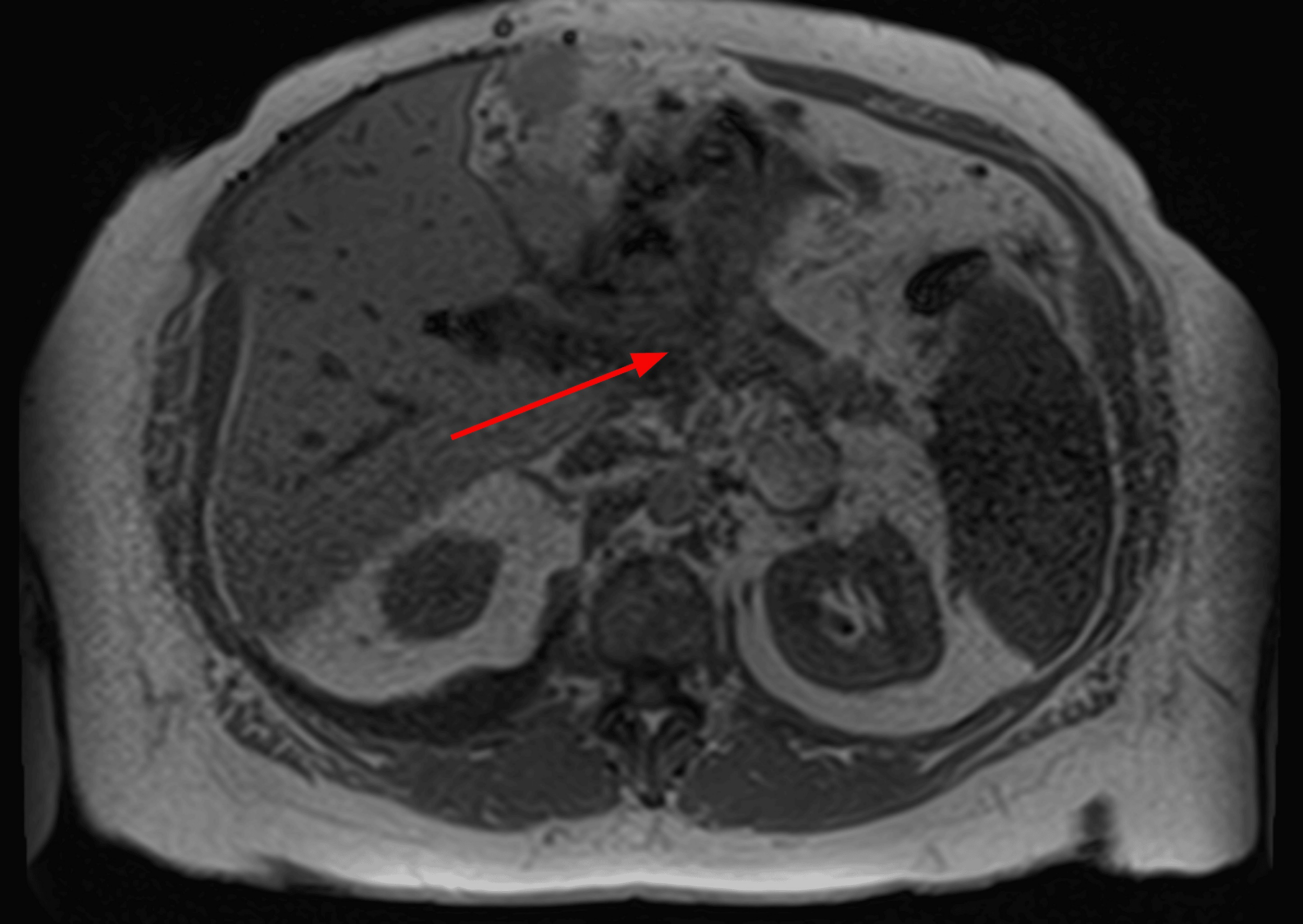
MRI of the abdomen and MRCP showing findings of sequelae of the patient's chronic history of pancreatitis. Chronic inflammatory changes of the pancreas. MRCP: Magnetic resonance cholangiopancreatography
